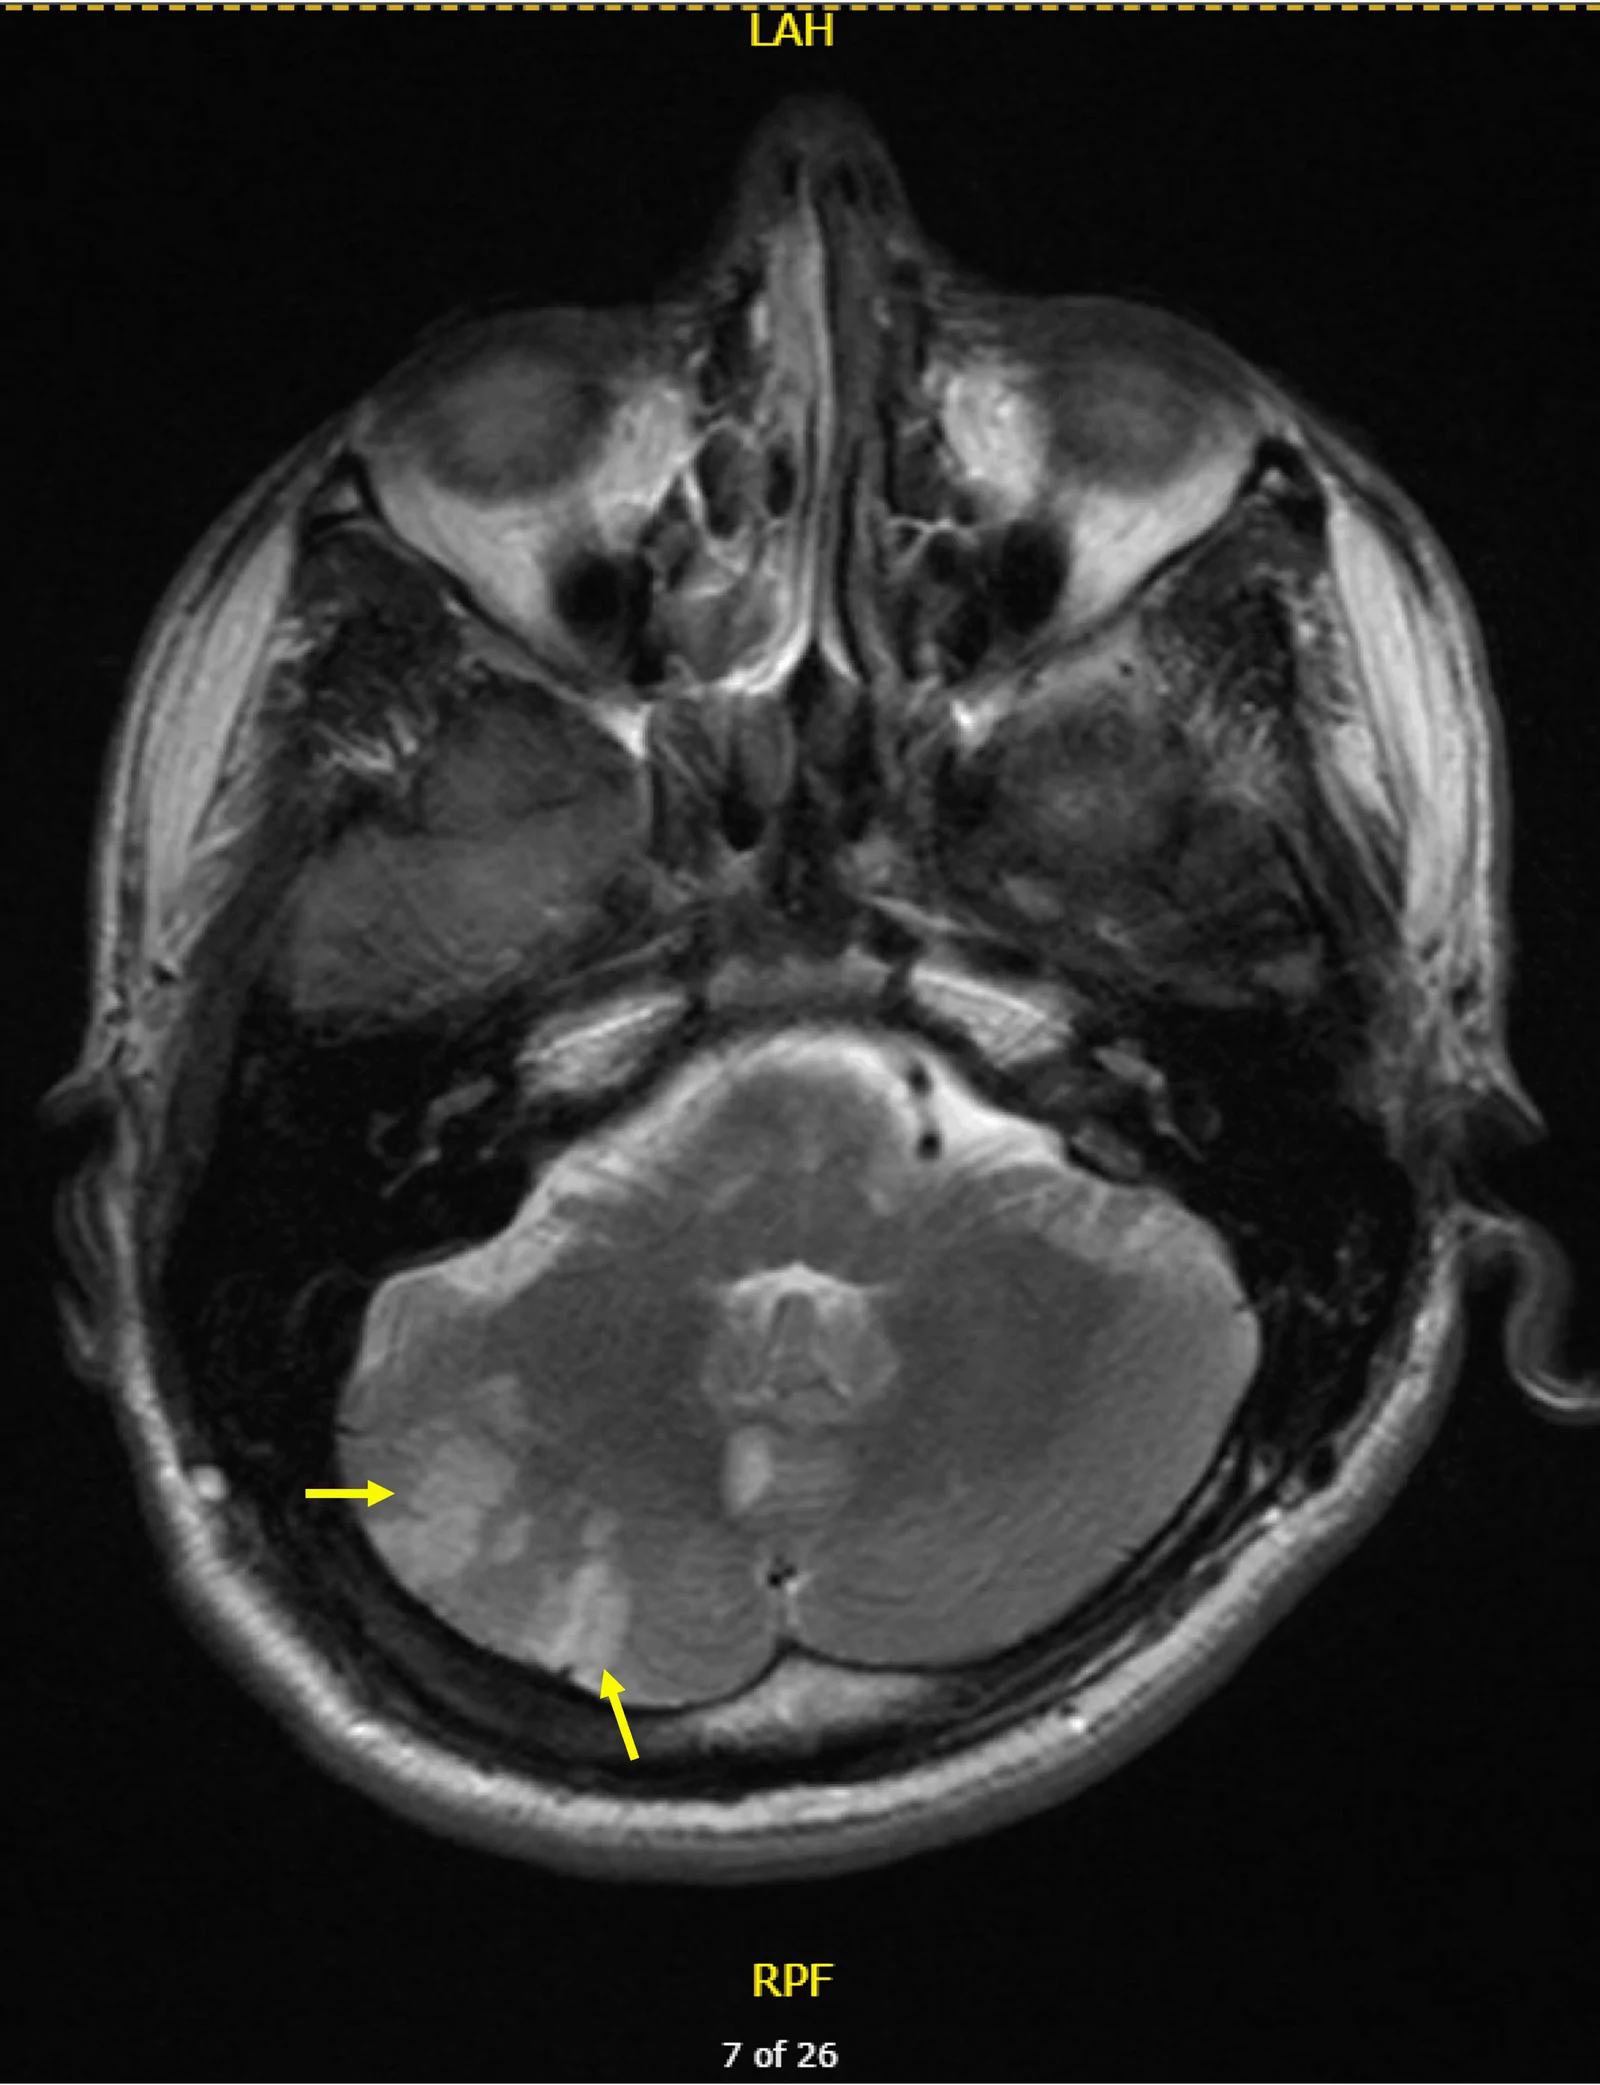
Day 5 MRI brain demonstrating acute infarct in the right cerebellar hemisphere Representative axial slice. Arrows point to areas of acute ischemic infarction within the right cerebellar hemisphere, a distinct process from the hemorrhagic findings in other imaging. This finding prompted a bubble-study echocardiogram and transesophageal echocardiogram, which identified a right-to-left shunt (patent foramen ovale).
